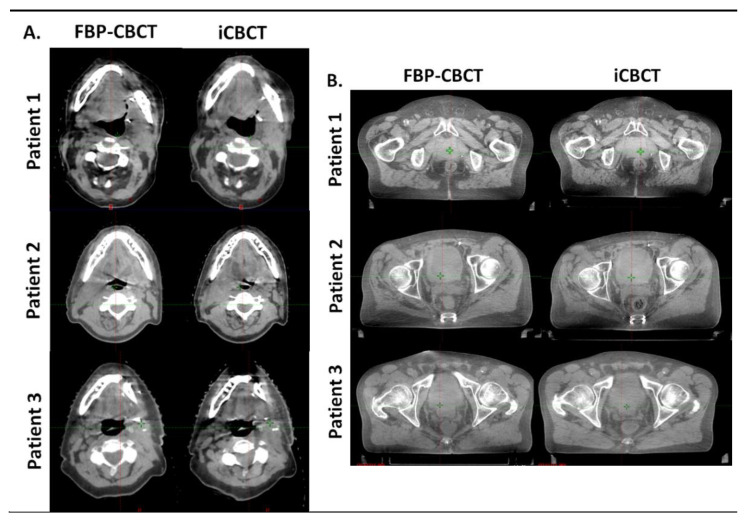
Comparison of head and neck (A) and pelvis (B) reconstruction with FBP (FBP-CBCT) and IR (iCBCT) on three patients. The series were matched on the same location and visualized with the same windowing.
